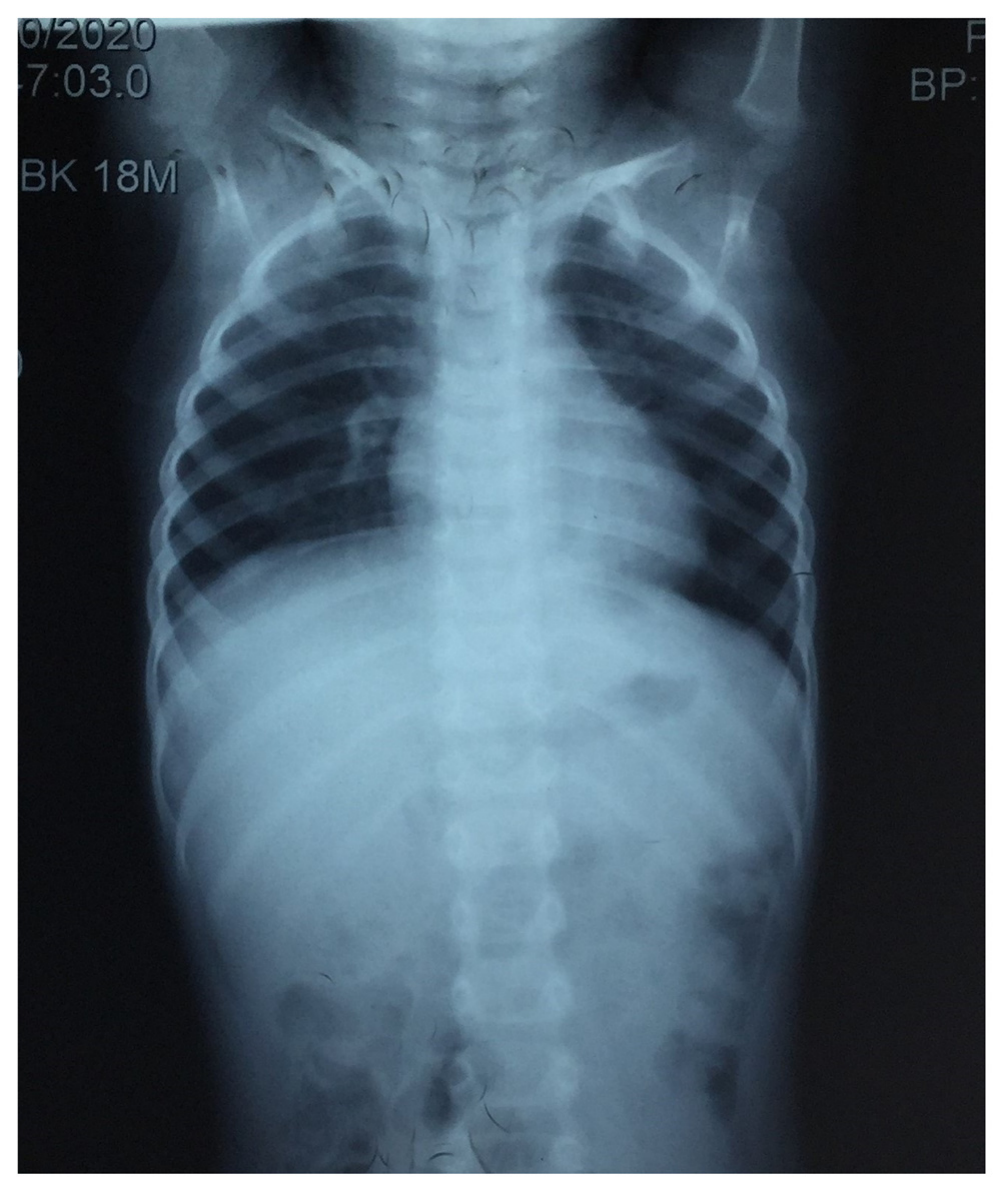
Chest radiograph showing normal bilateral lung fields, cardiophrenic and costophrenic angles, and cardiac shadow.
